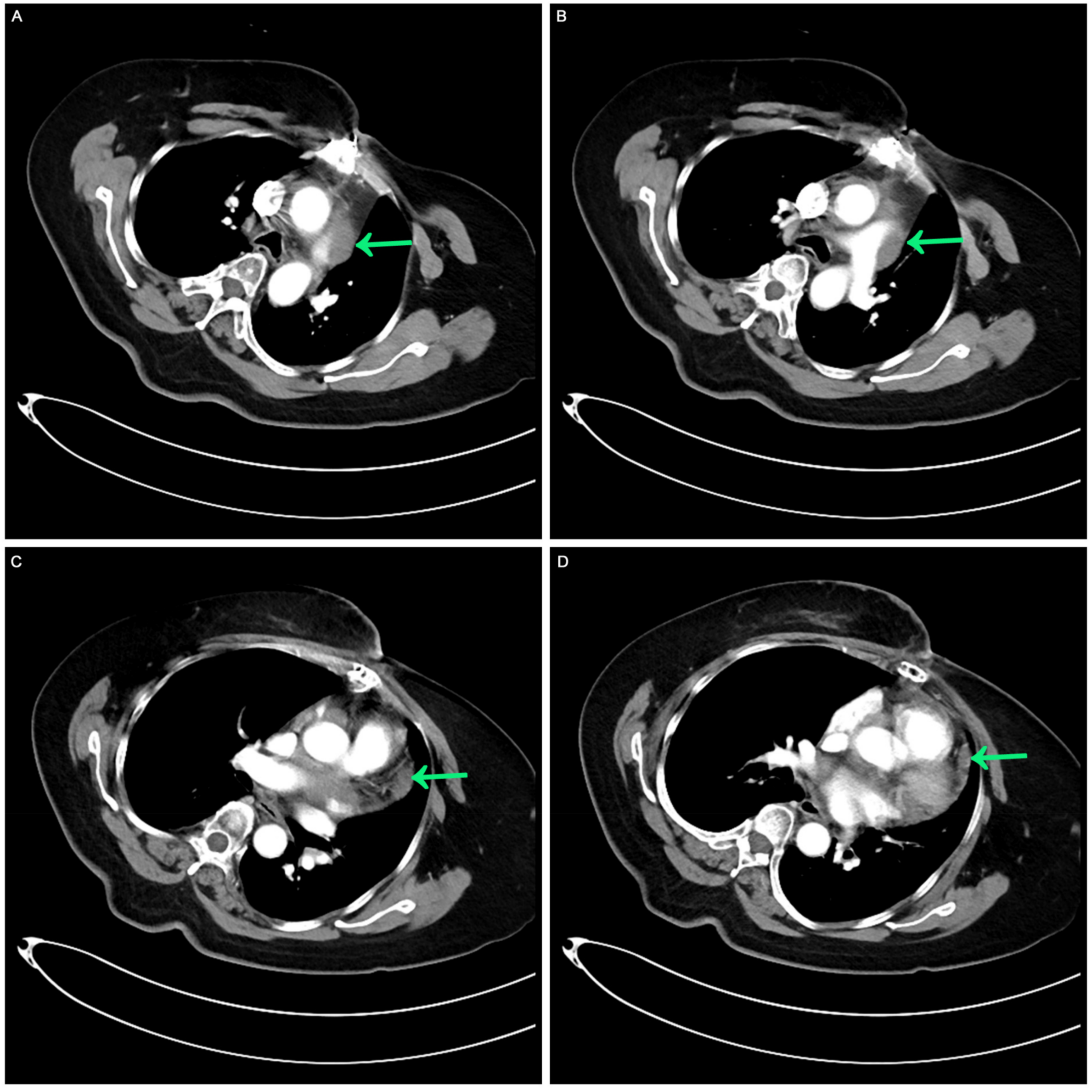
Contrast-enhanced CT after the completion of multimodal treatment. (A,B) The masses around the aorta and pulmonary arteries were retracted. (C,D) A comparable slight thickening of the pericardium was noted, receding from previous observations.
